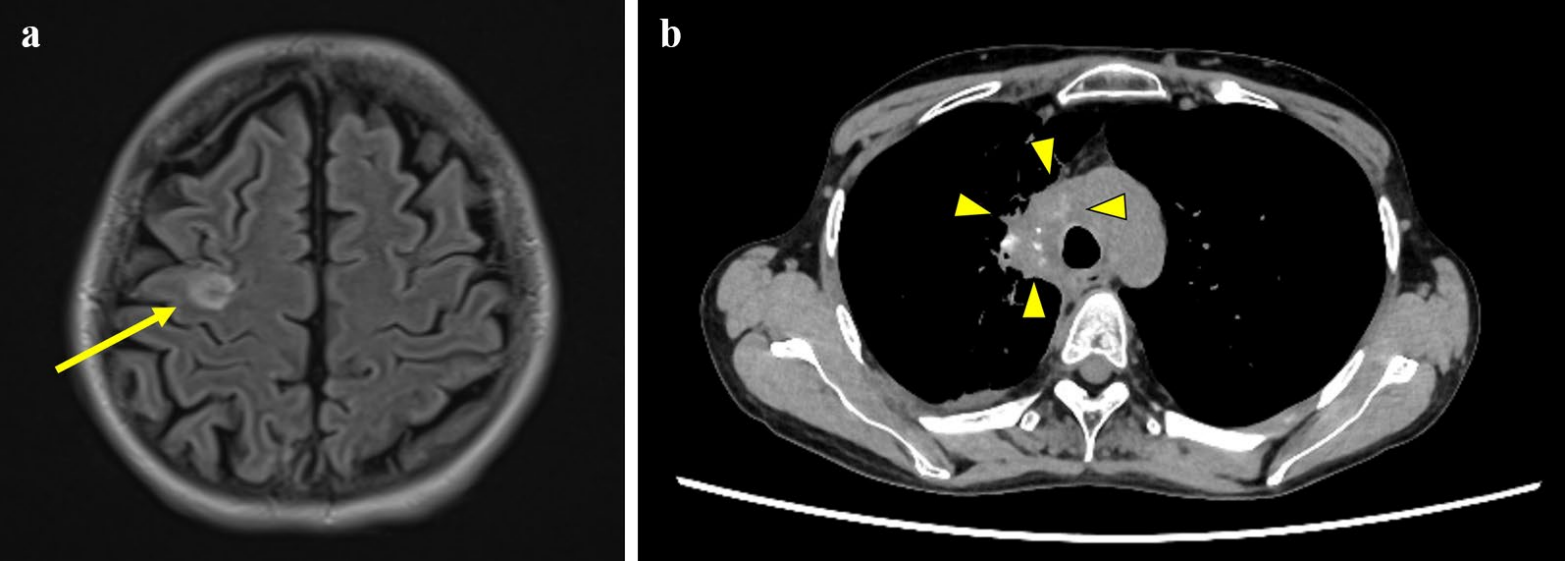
Follow-up imaging studies after surgery. a Brain MRI with FLAIR/T2WI (fluid attenuated inversion recovery/T2-weighted image) shows solitary metastasis in the right frontal lobe (arrow). b Chest computed tomography shows shrinkage of the primary lung tumor and mediastinal lymphadenopathy with an ill-defined boundary (arrowheads)
